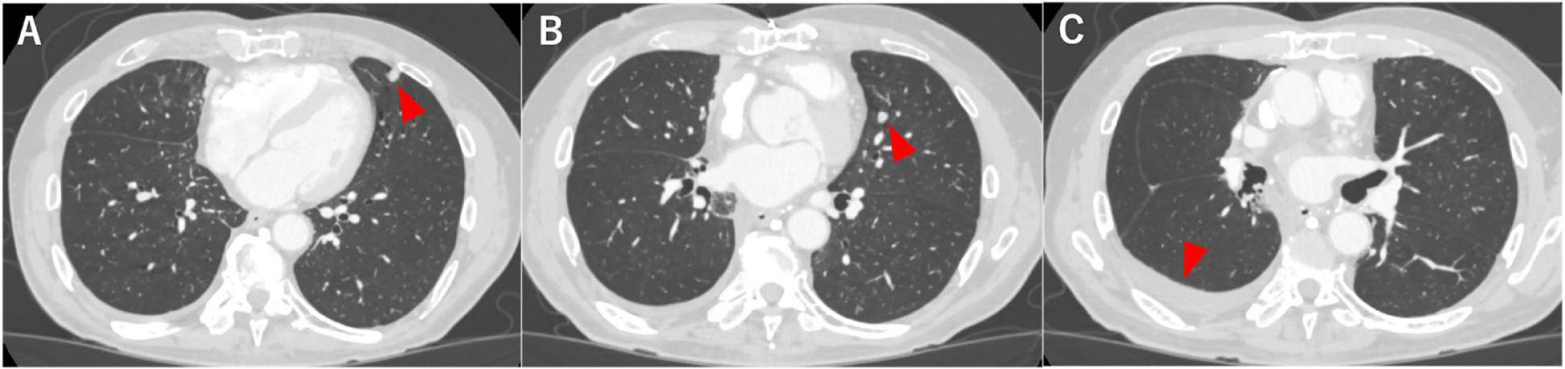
Contrast-enhanced chest CT image taken before the second surgery showing two solid nodules in the left lung lingular segment measuring 8 × 7 mm (A) and 7 × 5 mm (B), with a small amount of right pleural effusion (C)
